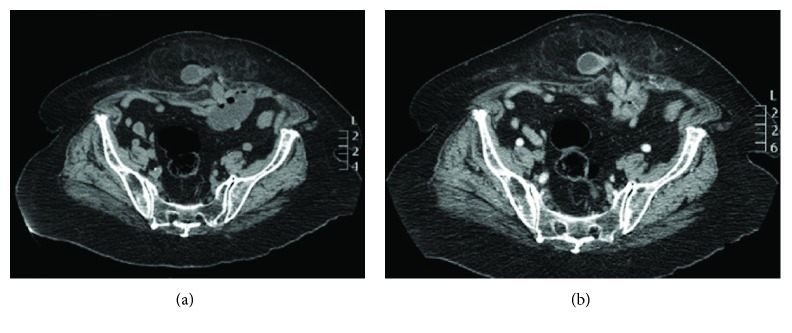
Axial CT scan of an 85-year-old female with abdominal pain. Unenhanced CT scan (a) shows an abdominal incisional hernia with protrusion of omental fat and a small bowel loop, the dilated afferent small bowel loop, and the collapsed efferent loop in the transition zone. Post contrast image in the portal venous phase (b) show normal enhancement of the small bowel loop within the hernia. However, the detection of the etiology of the bowel obstruction was already made on unenhanced CT.
